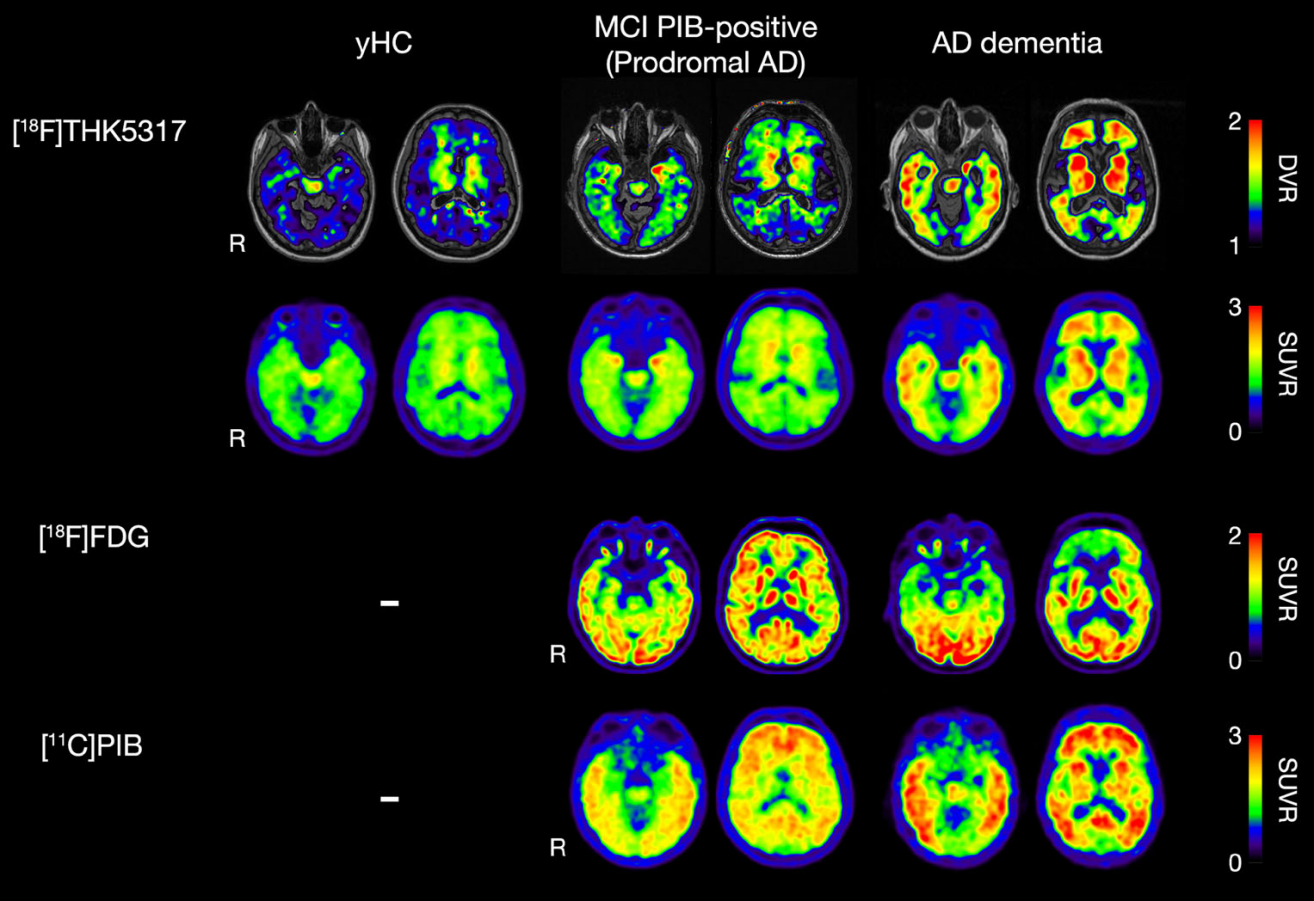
Sample [^18^F]THK5317 DVR, and [^18^F]THK5317, [^18^F]FDG and [^11^C]PIB SUVR images from a young healthy control (yHC), and patients with prodromal and dementia-stage Alzheimer’s disease (AD). DVR = distribution volume ratio; PIB = [^11^C]PIB; Prodromal AD = mild cognitive impairment (MCI) with [^11^C]PIB retention above the normal range (threshold standard uptake value ratio of 1.41); R = Right; SUVR = standard uptake value ratio
